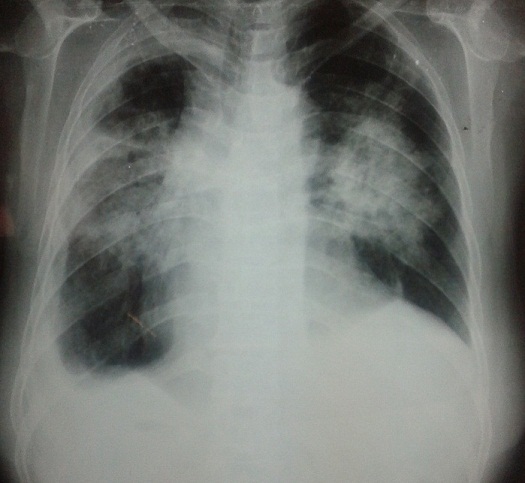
Chest X-ray revealing infiltrates in both lung fields
